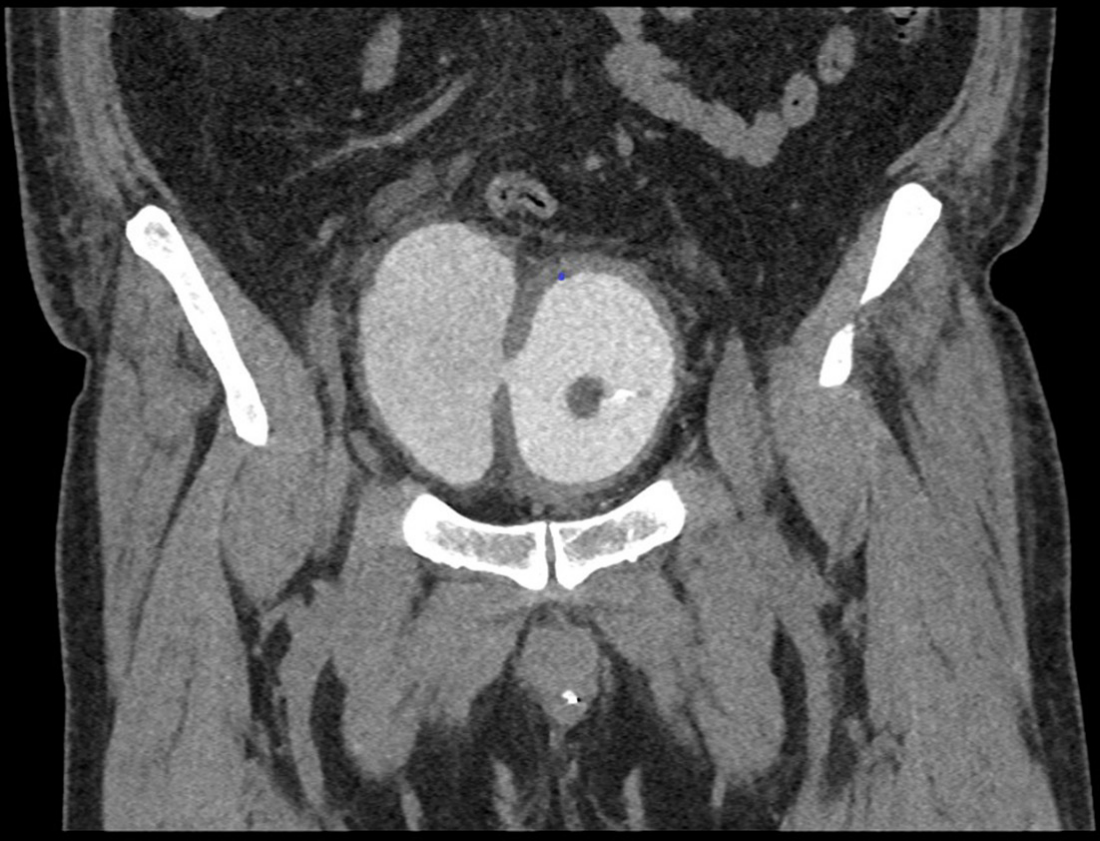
Second coronal delayed phase CT (Day 8 of admission), on soft tissue window
